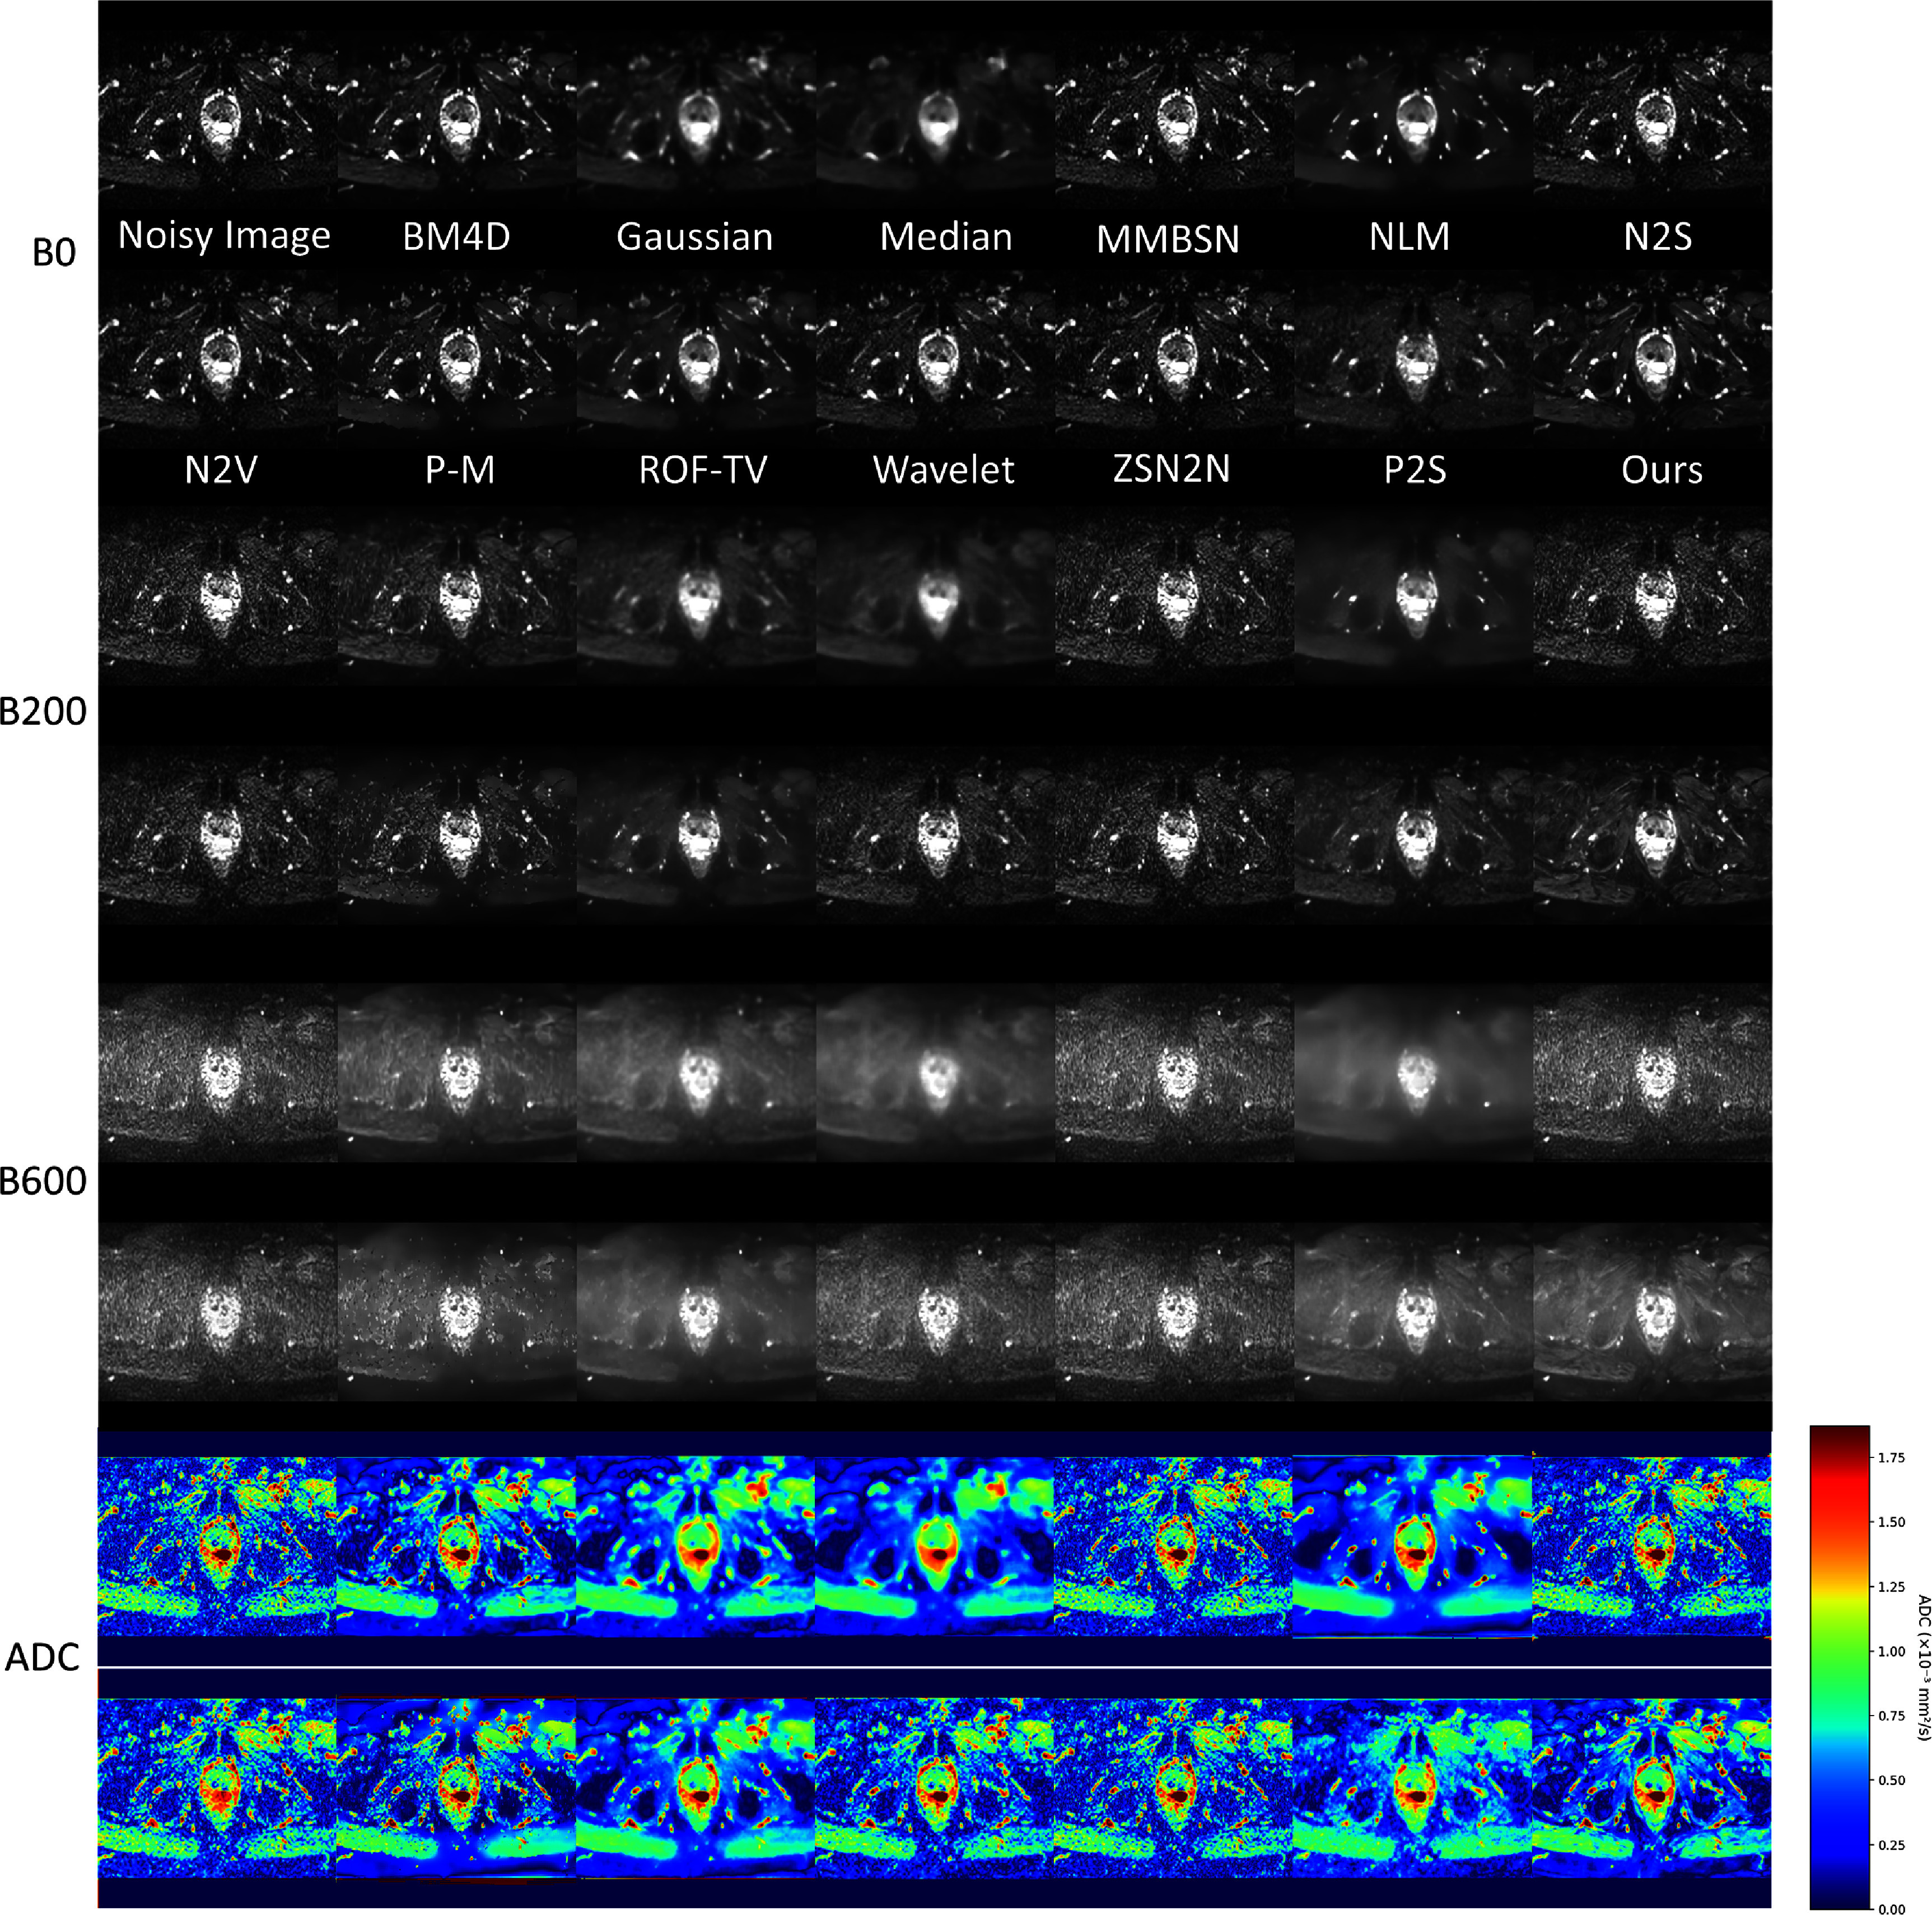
Comparison of pelvic DWI denoising results. In pelvic imaging with complex soft tissue structures, BL-INR effectively preserves tissue contrast while suppressing noise.
